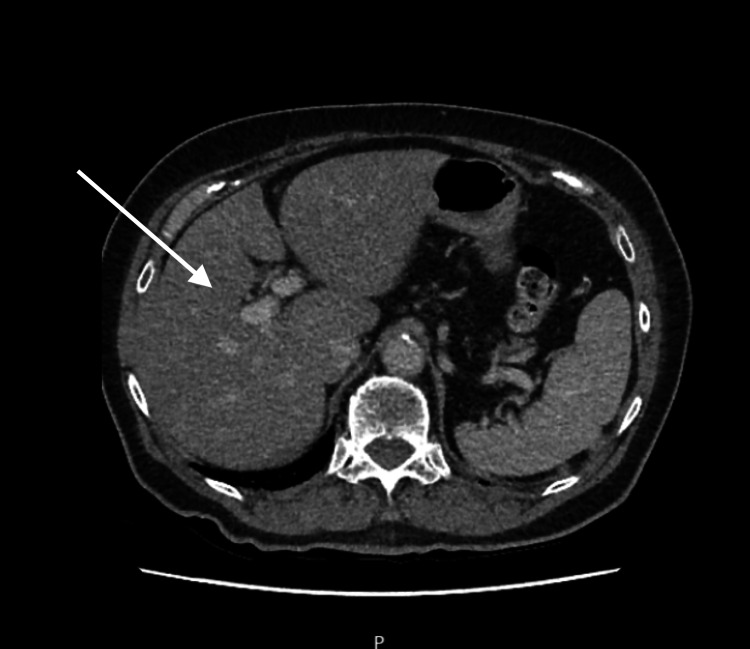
CT Abdomen and Pelvis with Contrast (CTAP): Post-Nephrectomy Findings Contrast-enhanced CT abdomen and pelvis demonstrates no acute intra-abdominal pathology. Postsurgical changes consistent with prior right nephrectomy are noted.
